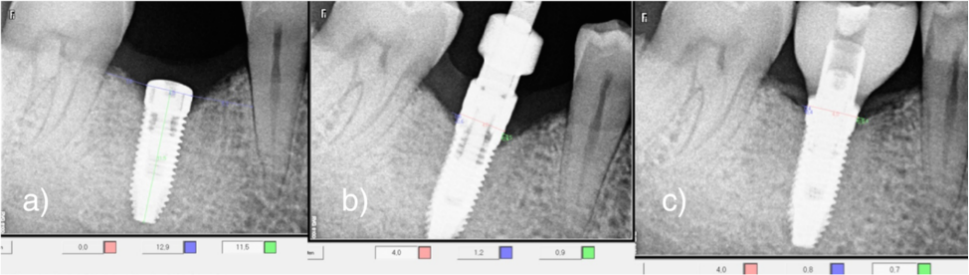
a–c Radiographic control a at time of implant placement (a), 3 months post-operative (b), and 6 months post-operative (c)
